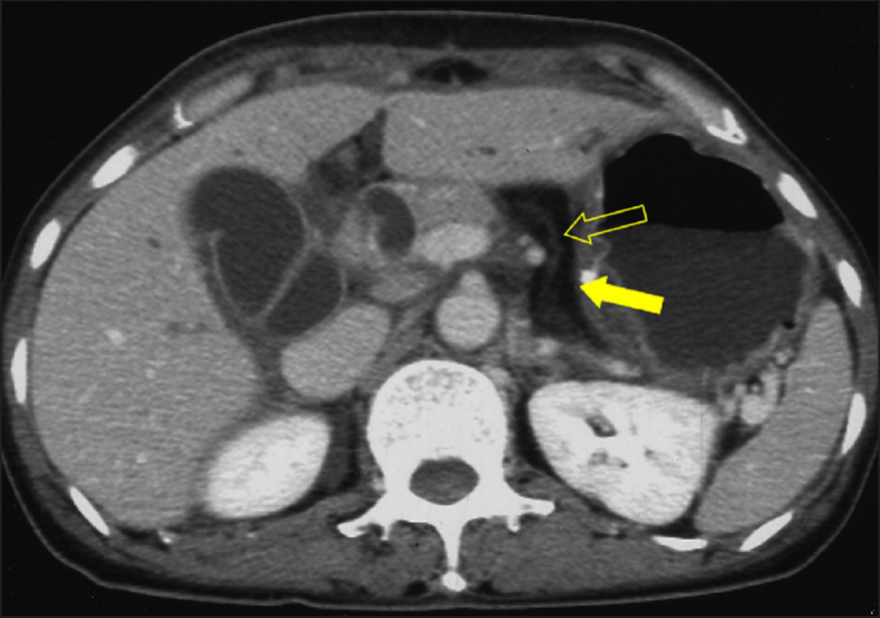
A 49-year-old woman with fatty replacement of distal pancreas. Axial portal venous phase CT image shows almost totally fatty change of distal pancreas (arrow). Note the pancreatic duct (open arrow) within the fat density pancreas tissue.
